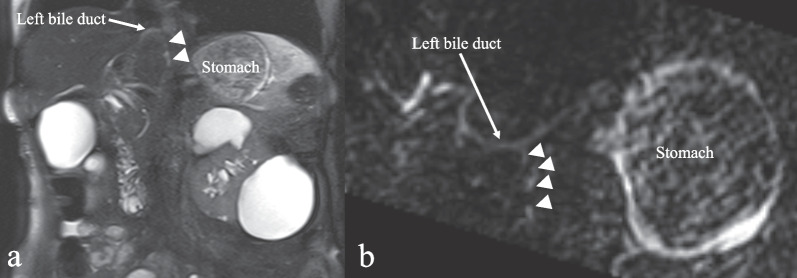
Magnetic resonance imaging. A variant bile duct originating from the left hepatic duct and proceeding to the stomach (arrow heads) was recognized 1 month after the operation (a T2-weighted image, b diffusion-weighted image)
